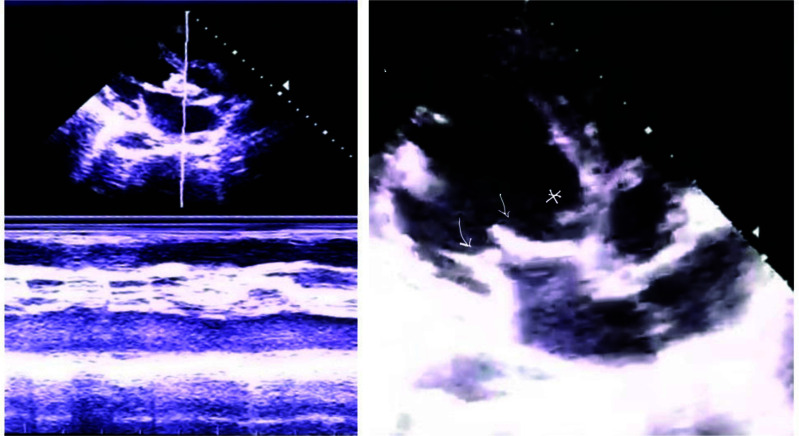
TTE- Long axis parasternal view- in left image M mode shows dense and shaggy echoes on aortic valve (Ao) suggestive of endocarditis with thickened aortic valve and right image shows aortic(asterix) and mitral (arrow) vegetations.
